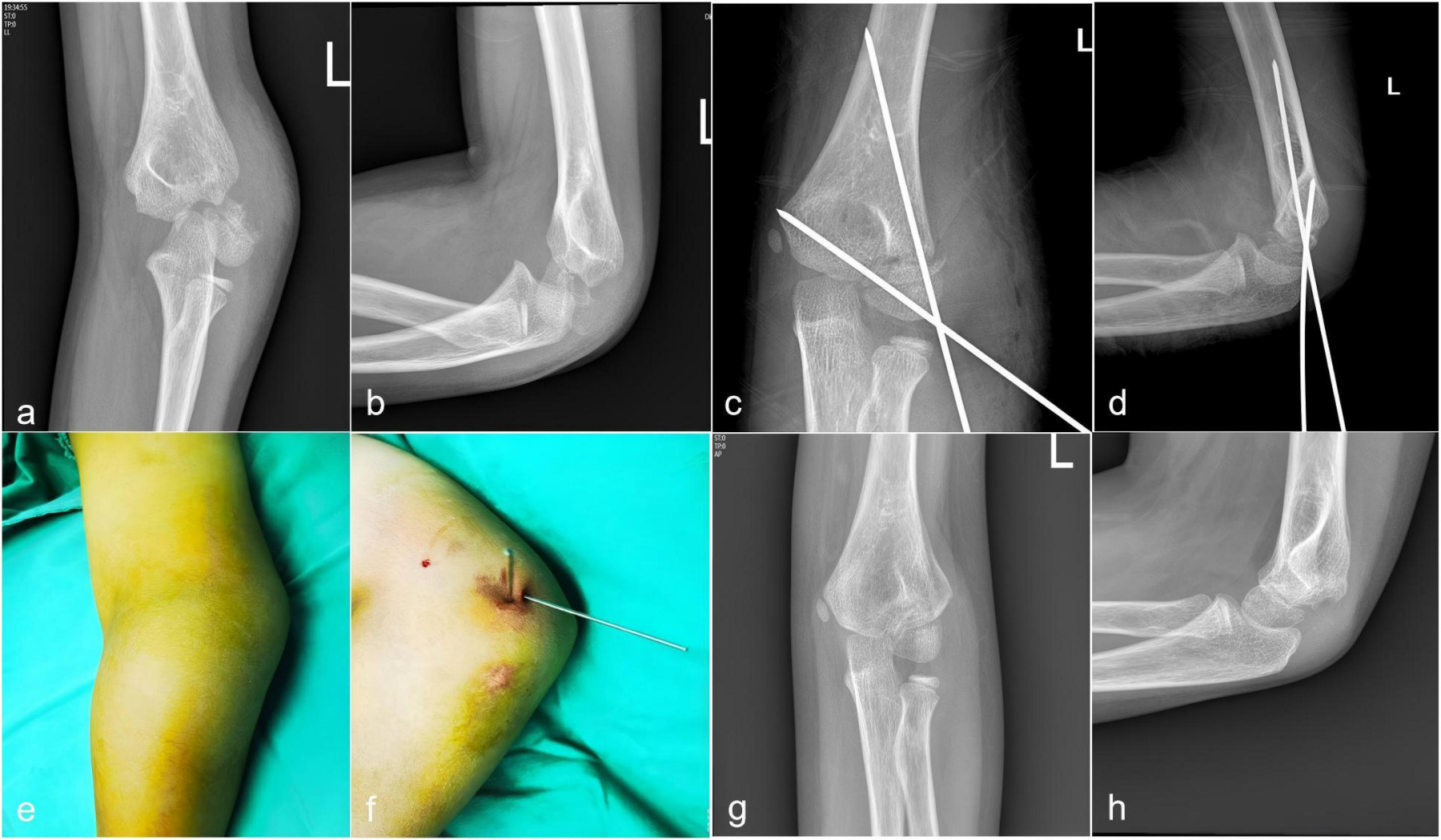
Sequential imaging and clinical appearance of a representative case of Song V lateral humeral condyle fracture treated with closed reduction percutaneous pinning. (a,b) Preoperative anteroposterior and lateral radiographs showing complete displacement and rotation of the lateral condylar fragment. (c,d) Intraoperative radiographs after successful closed reduction and Kirschner wire placement. (e,f) Clinical photographs of the elbow following percutaneous pinning, demonstrating minimal surgical wound. (g,h) Follow-up AP radiograph at 12 months postoperatively, showing complete fracture union without evidence of avascular necrosis or significant deformity.
